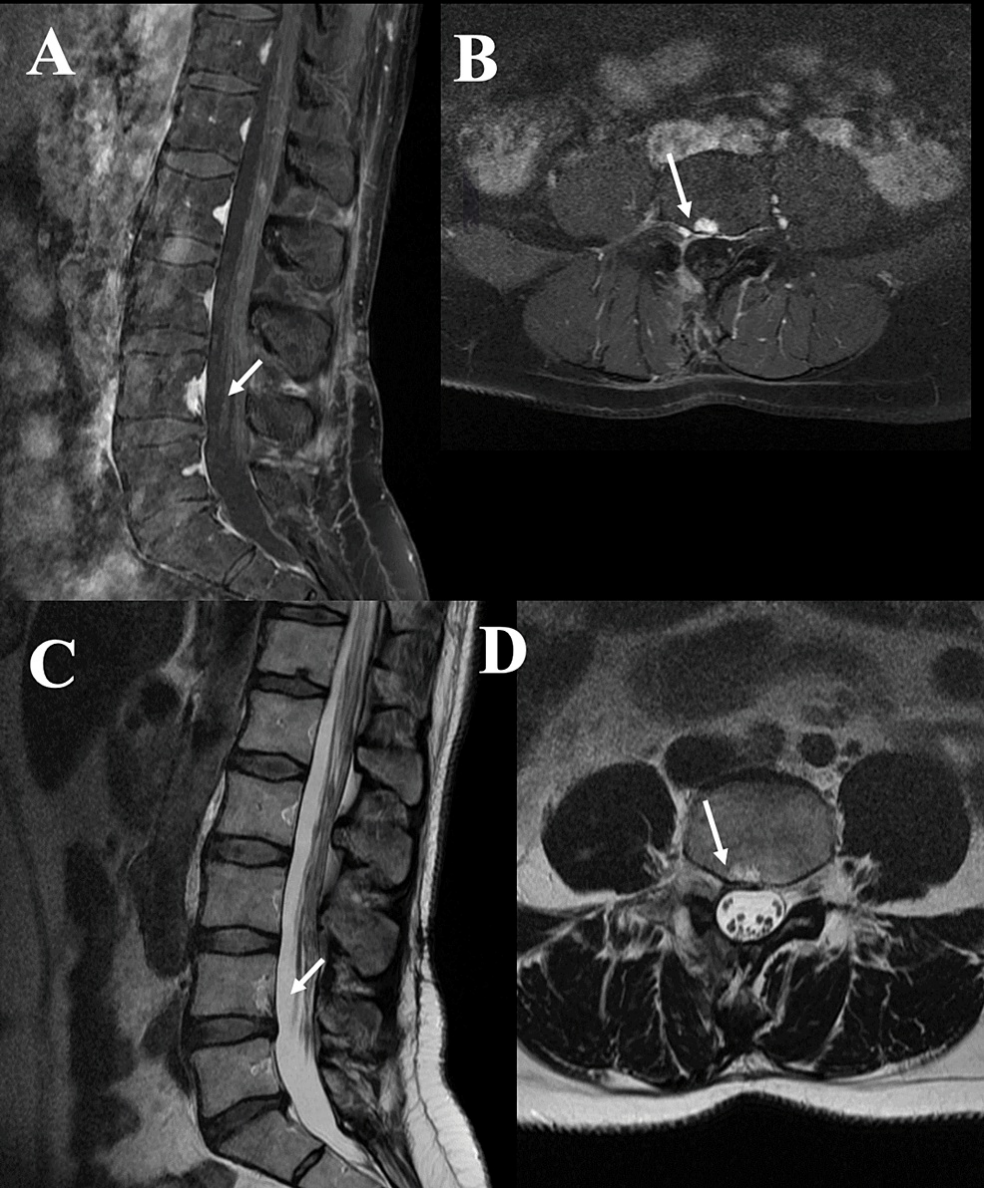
Postoperative radiological findings. Six months postoperative T1-weighted contrast enhanced sagittal (A) and axial (B) as well as T2-weighted sagittal (C) and axial (D) MRI scans demonstrated completely resection of the lesion and radicular decompression.
